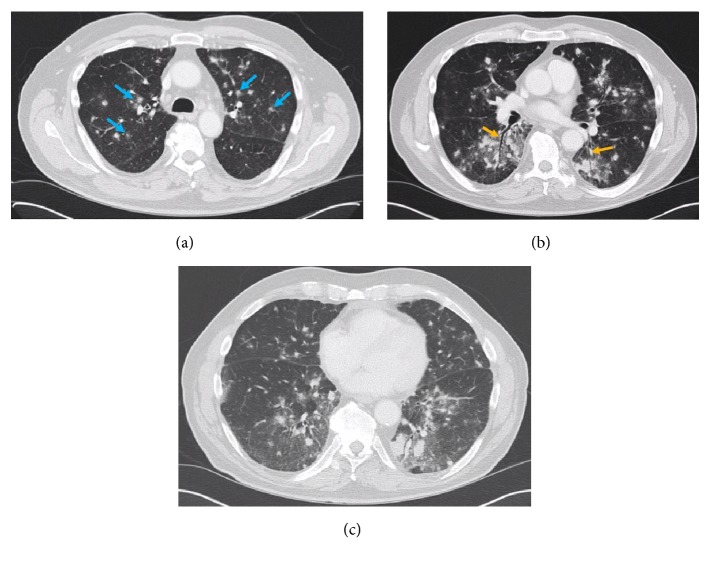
Axial lung window CT slices (a, b, c) at different levels demonstrate innumerable ill-defined solid nodules with peripheral ground-glass halos in a peribronchovascular distribution (blue arrows) and more prominent in the left lung. Air-bronchograms were seen (orange arrows).
